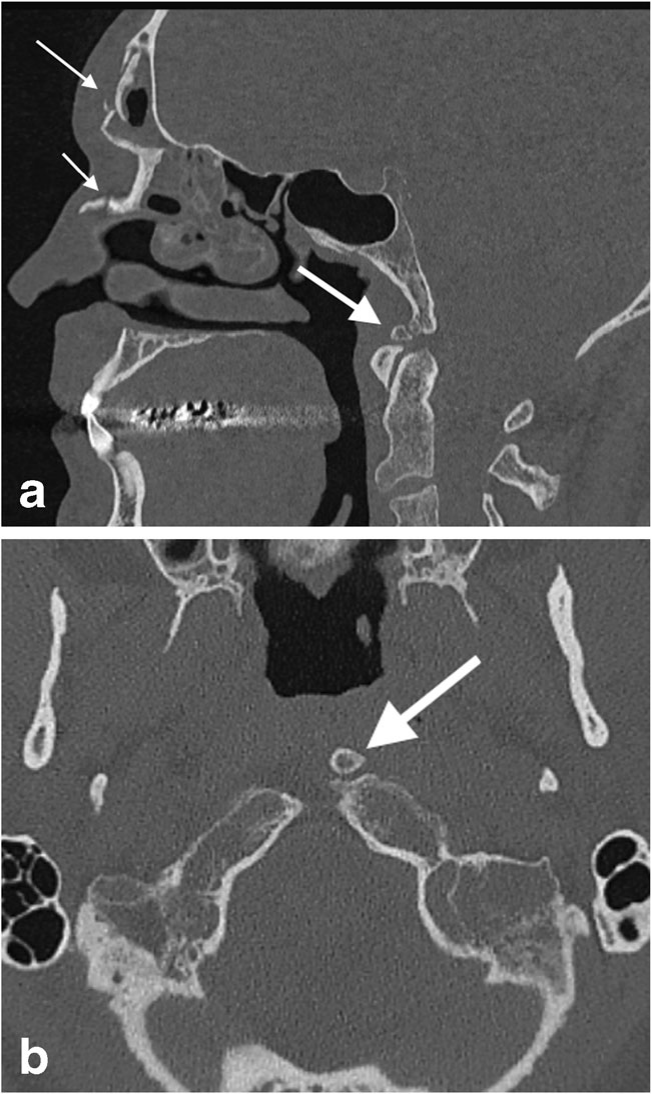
Condylus tertius: a sagittal reformatted CT image through the craniocervical junction of a 54-year-old male cyclist who fell of his bicycle at speed sustaining facial fractures. The condylus tertius of unfused hypochordal bow is demonstrated (*thick arrow*) and is an important mimic of fracture at the basion. Note the depressed comminuted facial fractures involving the frontal sinus and nasal bone (*thin arrow*s); b axial CT image of the same patient demonstrates the unfused bone remnant at the left side of the basion (*arrow*), not to be mistaken for a displaced fracture fragment
